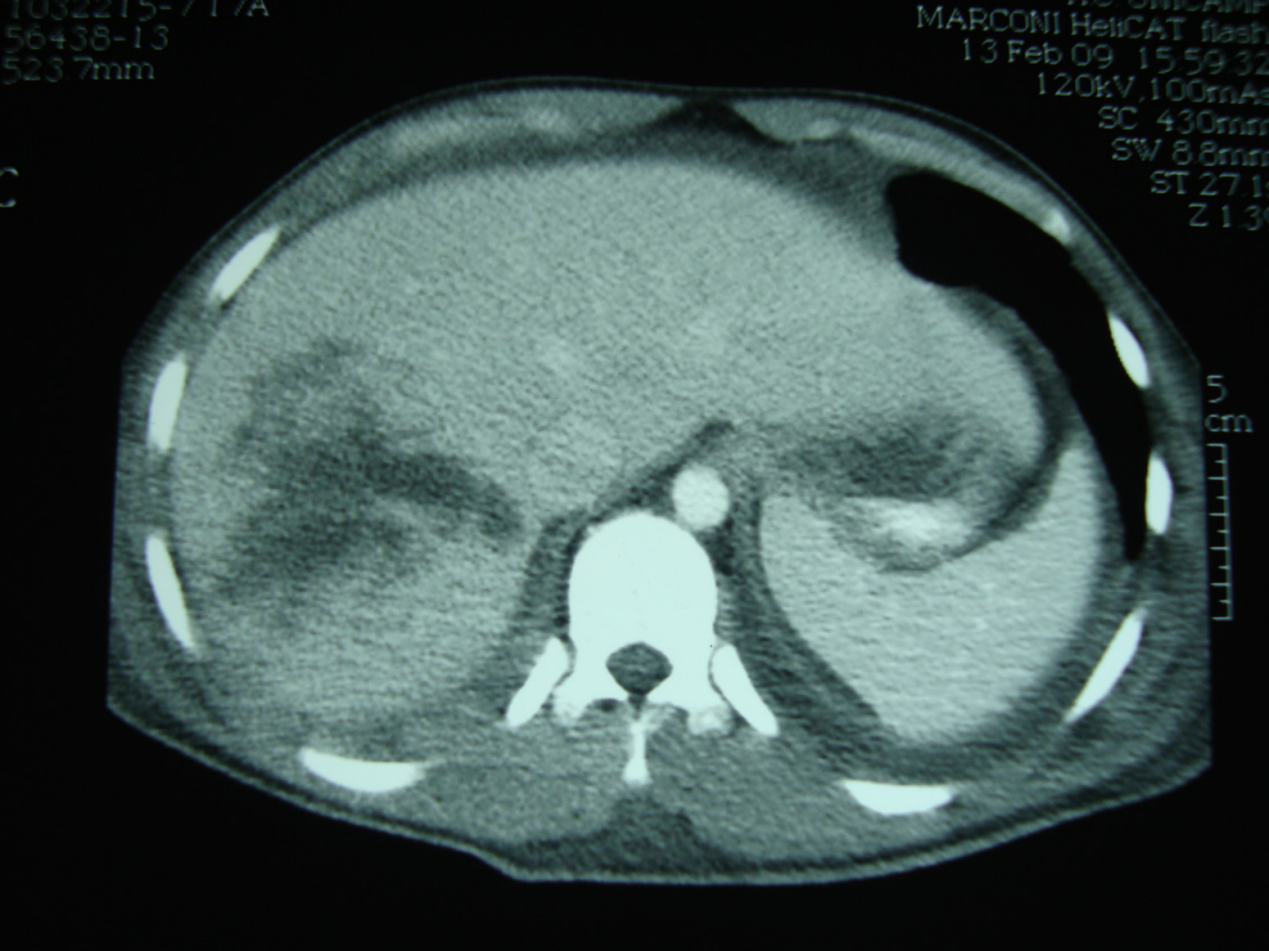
Pedestrian hit by a car; multislice CT showing abdominal free fluid and intraparenchymal hematoma in the right lobe (grade IV hepatic injury), no blush of contrast in the arterial phase.
